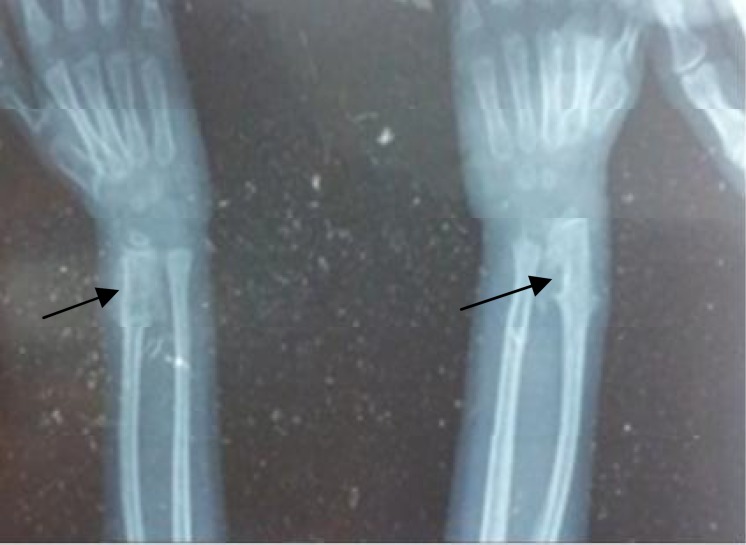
Radiograph of the wrist joint showing exostoses *crests*
